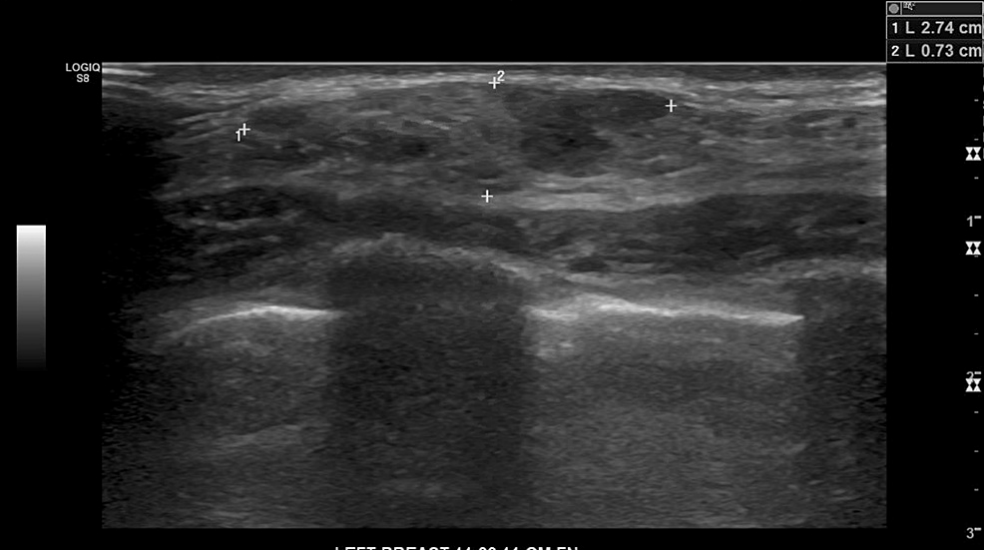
Ultrasound Ultrasound findings stated that "The 27 mm x 16 mm x 10 mm lobulated mass in the left breast at 11 o'clock appears suspicious of malignancy."
